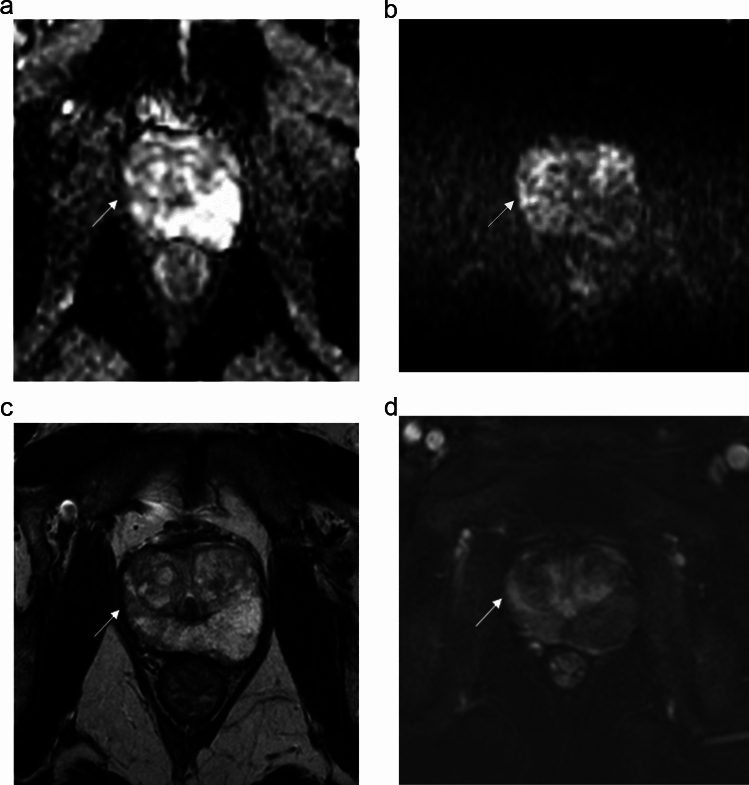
Case of Likert scoring by reader 1 reinforcing the level of suspicion of PI-RADS categorization in a 53-year-old man with a prostate-specific antigen level density of 0.08 ng/mL/mL and negative digital rectal examination. The index lesion in the right anterior peripheral zone of the midgland showed wedge-shaped mild hypointensity on the apparent diffusion coefficient map (arrow in a) and wedge-shaped mild hyperintensity on b = 2000s/mm^2^ image (b), slight hypointensity on T2-weighted imaging (arrow in c) and early focal enhancement after contrast administration (arrow in d). The lesion was assessed as PI-RADS 2 and Likert 2. Transperineal systematic biopsy cores in the same quadrant and adjacent quadrant showed gland atrophy/subatrophy and chronic prostatitis
